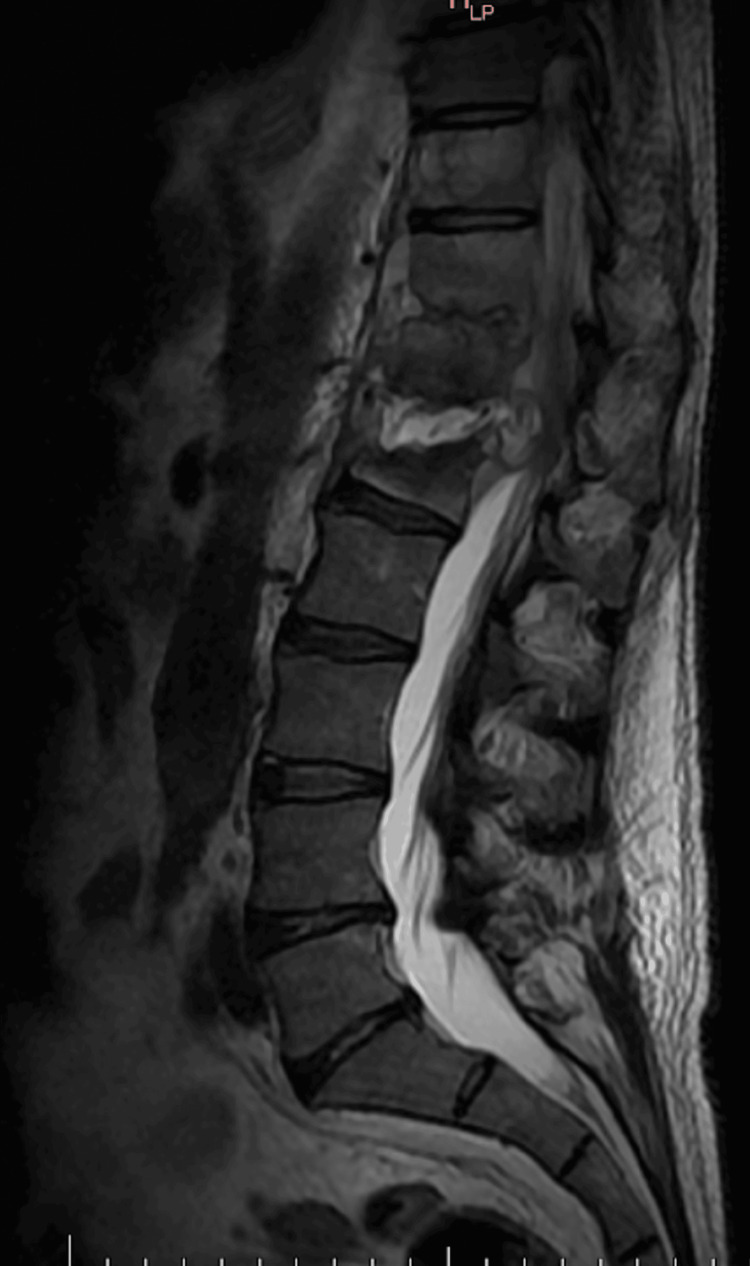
MRI T2-weighted sequence. High-intensity signal inside the L1-L2 disk on T2-weighted sequences and destroyed L1 vertebral end plate, accompanied with high-signal-intensity marrow edema. Additionally, a left psoas muscle abscess was noted.
